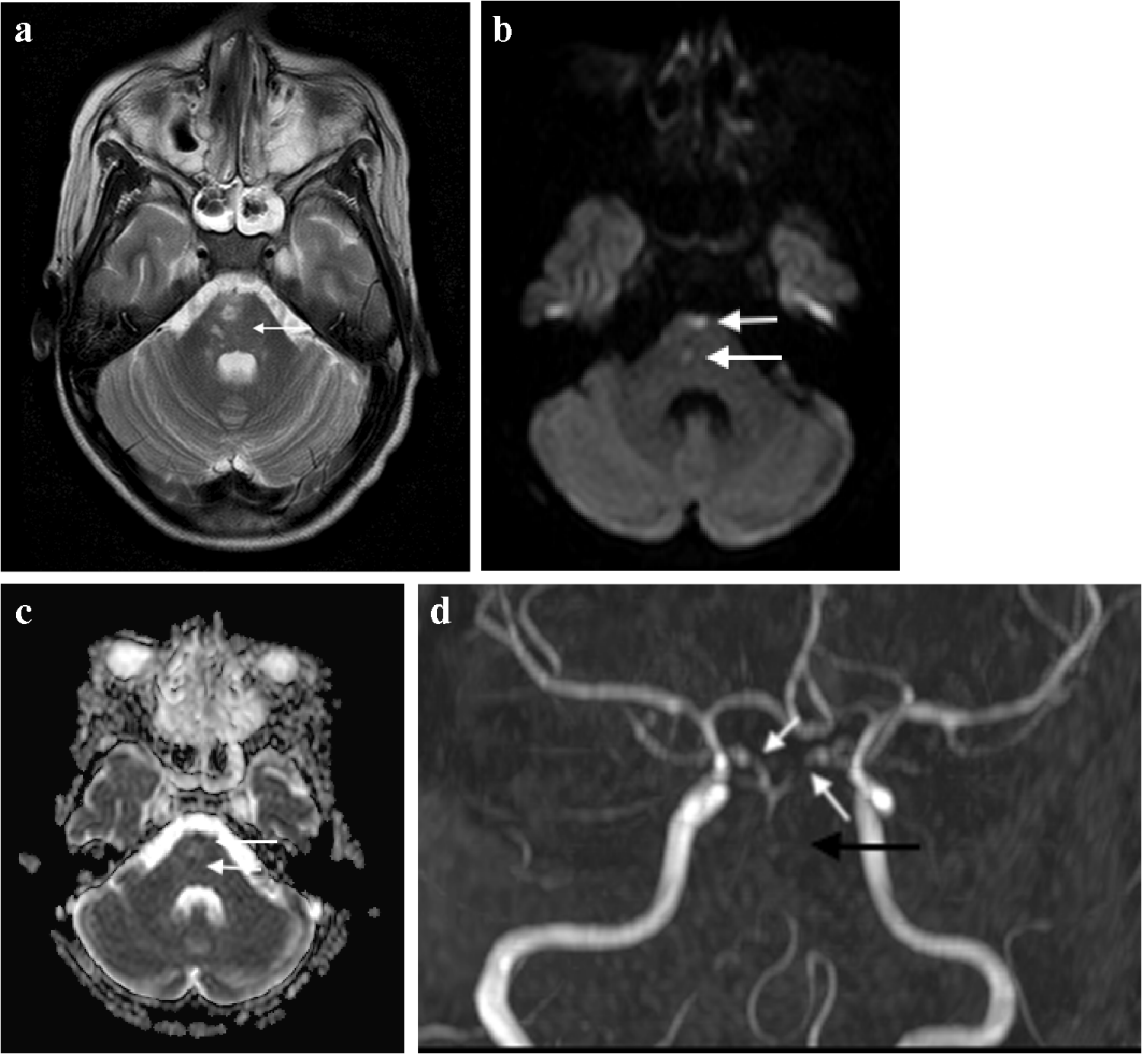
Magnetic resonance imaging of the brain in a 12-year-old boy with tuberculous meningitis and persistent decreased level of consciousness. a Axial T2 demonstrates multiple hyperintense foci in the pons (*arrow*). b Axial diffusion-weighted image demonstrates restricted diffusion (*arrow*) c Low signal (*arrows*) on the apparent diffusion coefficient map confirms infarcts. d Coronal magnetic resonance angiogram image shows partial occlusion of the basilar artery (*black arrow*) and irregularity of both posterior communicating arteries (*white arrows*)
